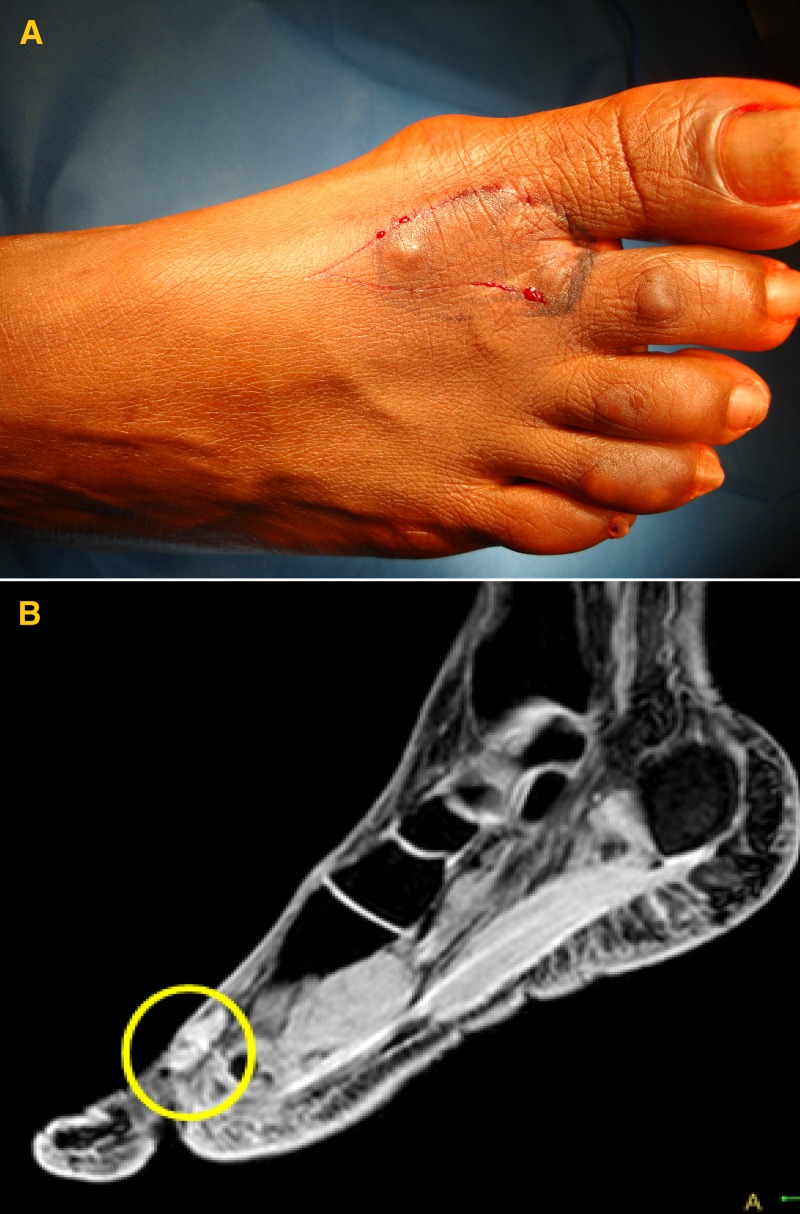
(A) Nodular lesion located on the right foot. (B) Magnetic resonance imaging (MRI) showed two elongated nodules in subcutaneous fat without bone or tendon involvement.
